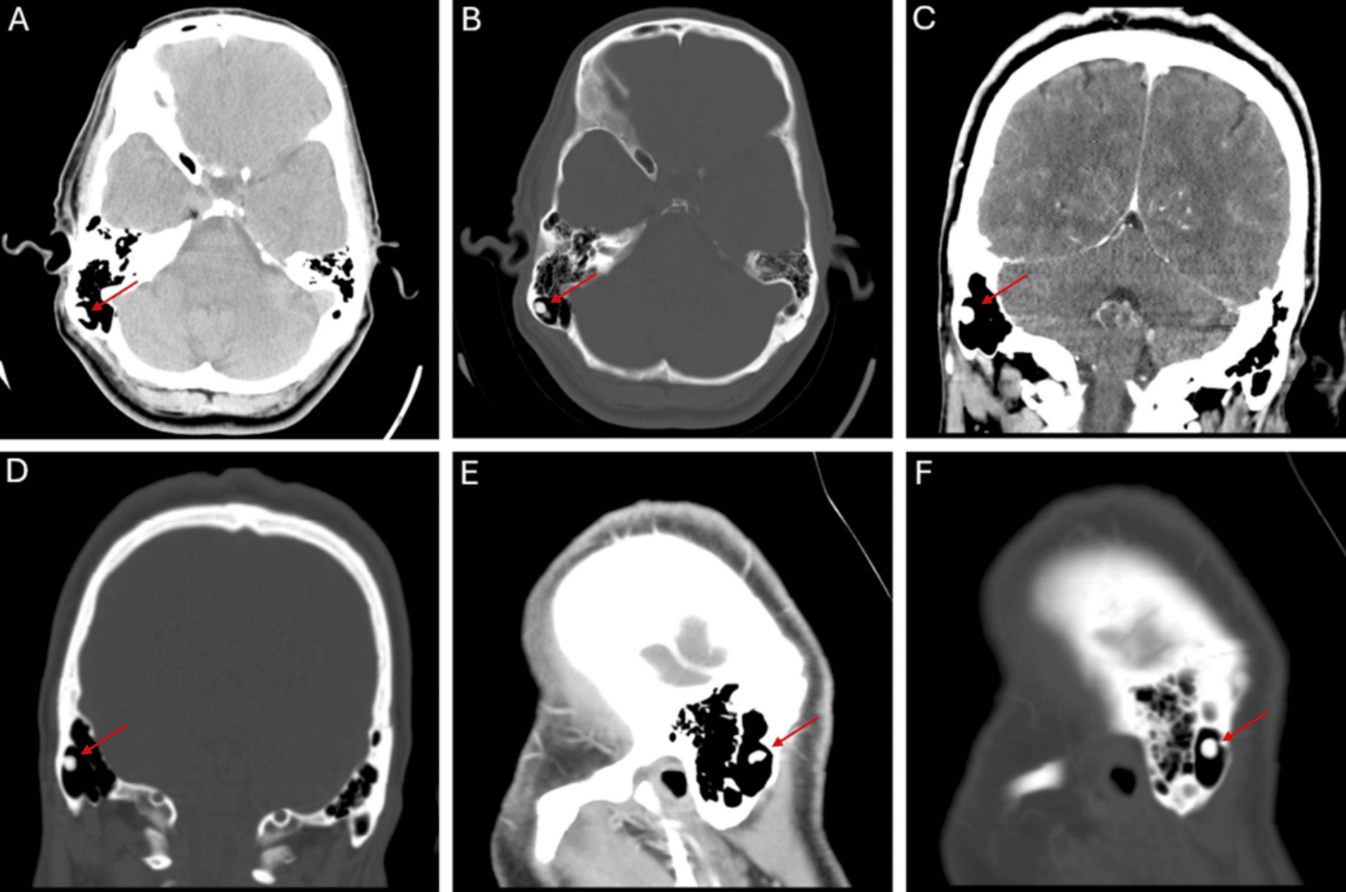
Enhanced and non-enhanced CT of the head showing a calcified nodule with a surrounding pneumatized lucent lesion in the right mastoid air cells. (A,B) Axial, (C,D) coronal, and (E,F) sagittal views are shown.
